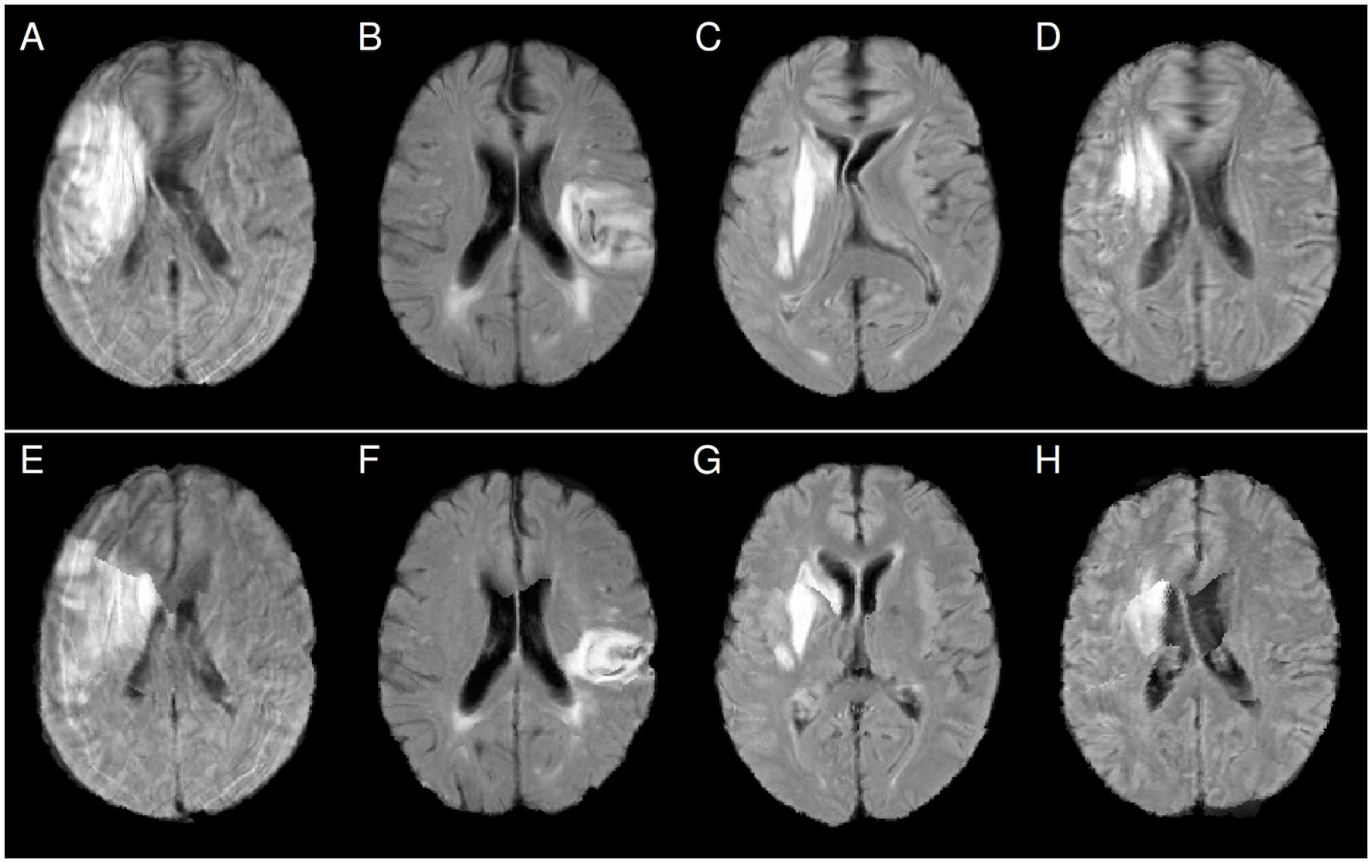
Comparison of FLAIR patient images after normalization using nonlinear FLAIR patient data to atlas registration (panels A–D) and nonlinear T1-weighted patient data to atlas registration (panels E–H). Distortions like frontal blobs and midline shifts after normalization are clearly reduced after using the T1-weighted data.
